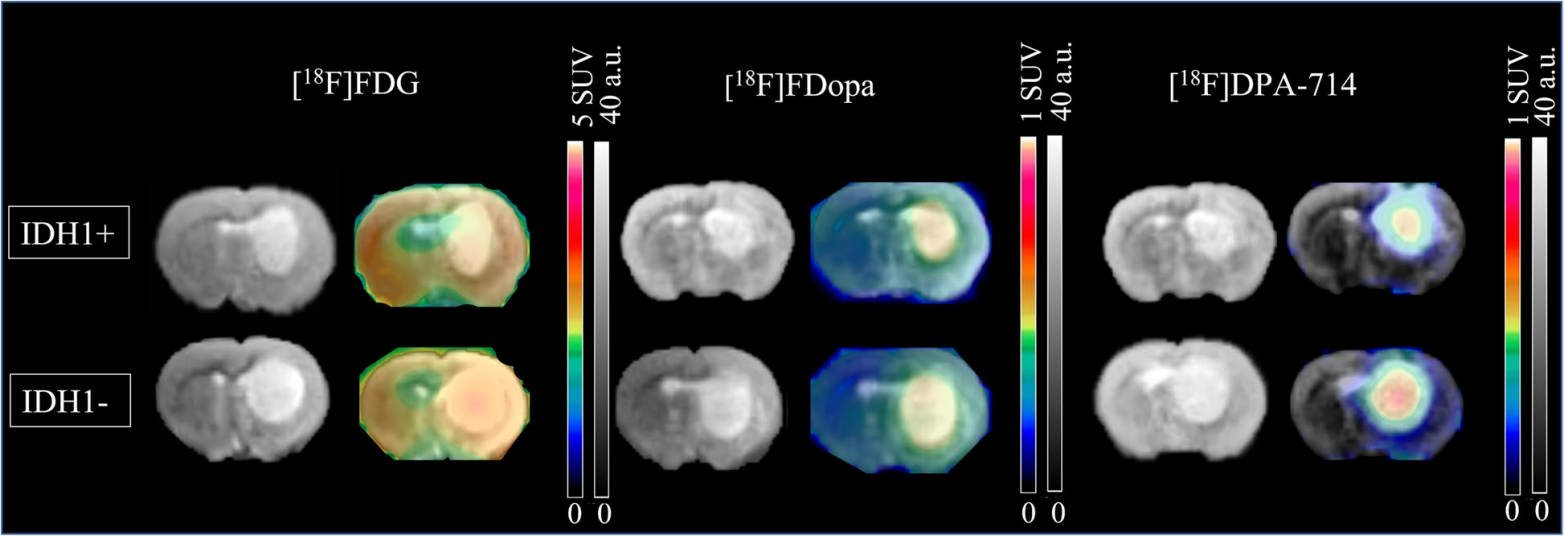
Representative axial images of T_2_-w MRI and static PET imaging merged with T_2_-w MRI for IDH1+ and IDH1- tumors with [^18^F] FDG, [^18^F] FDopa and [^18^F]DPA-714. MRI is expressed as signal intensity and PET on an SUV scale
